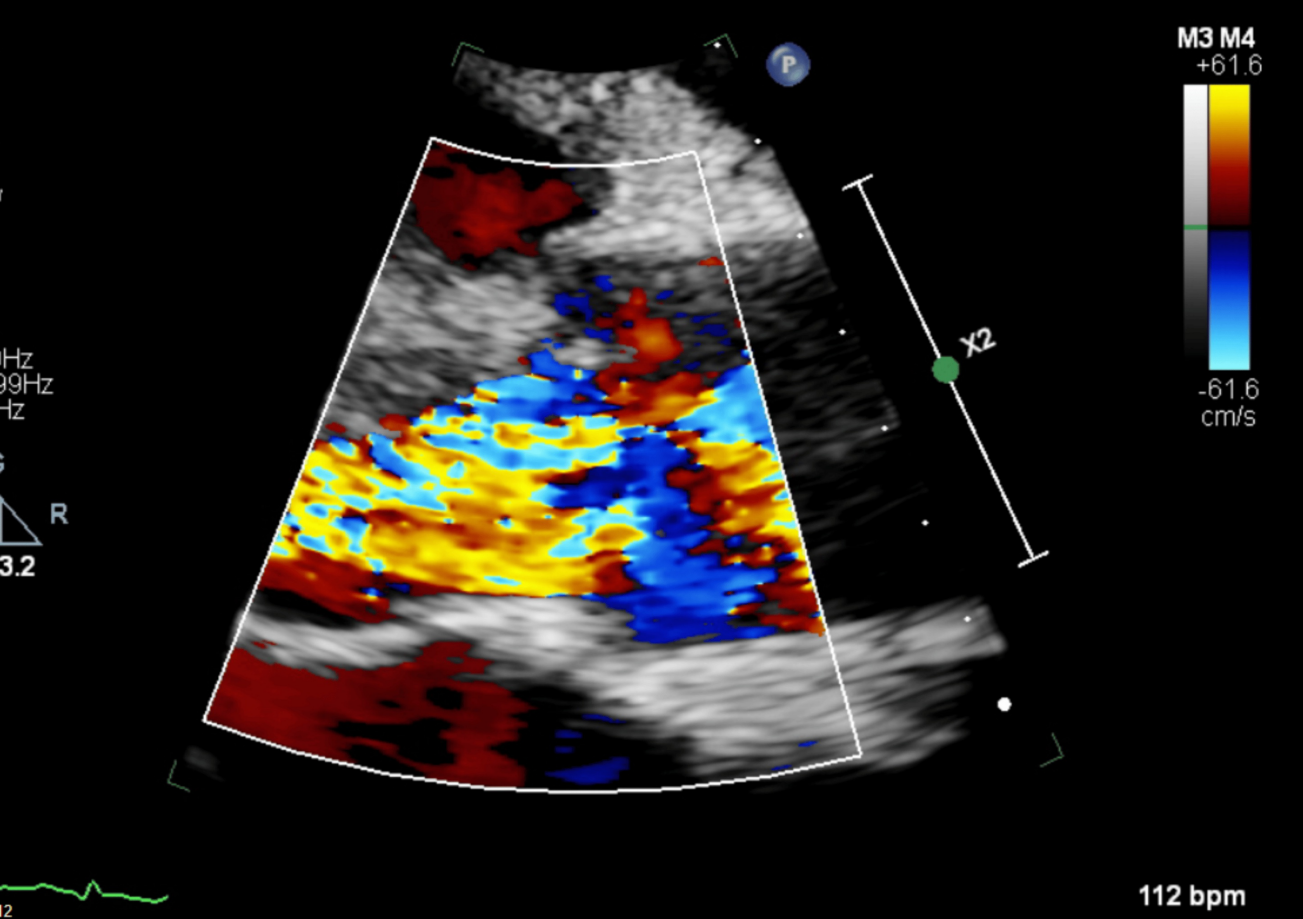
TTE PLAX view - zoom in on the aortic valve with colour flow mapping showing the aortic regurgitation TTE: Transthoracic echocardiogram, PLAX: Parasternal long axis
